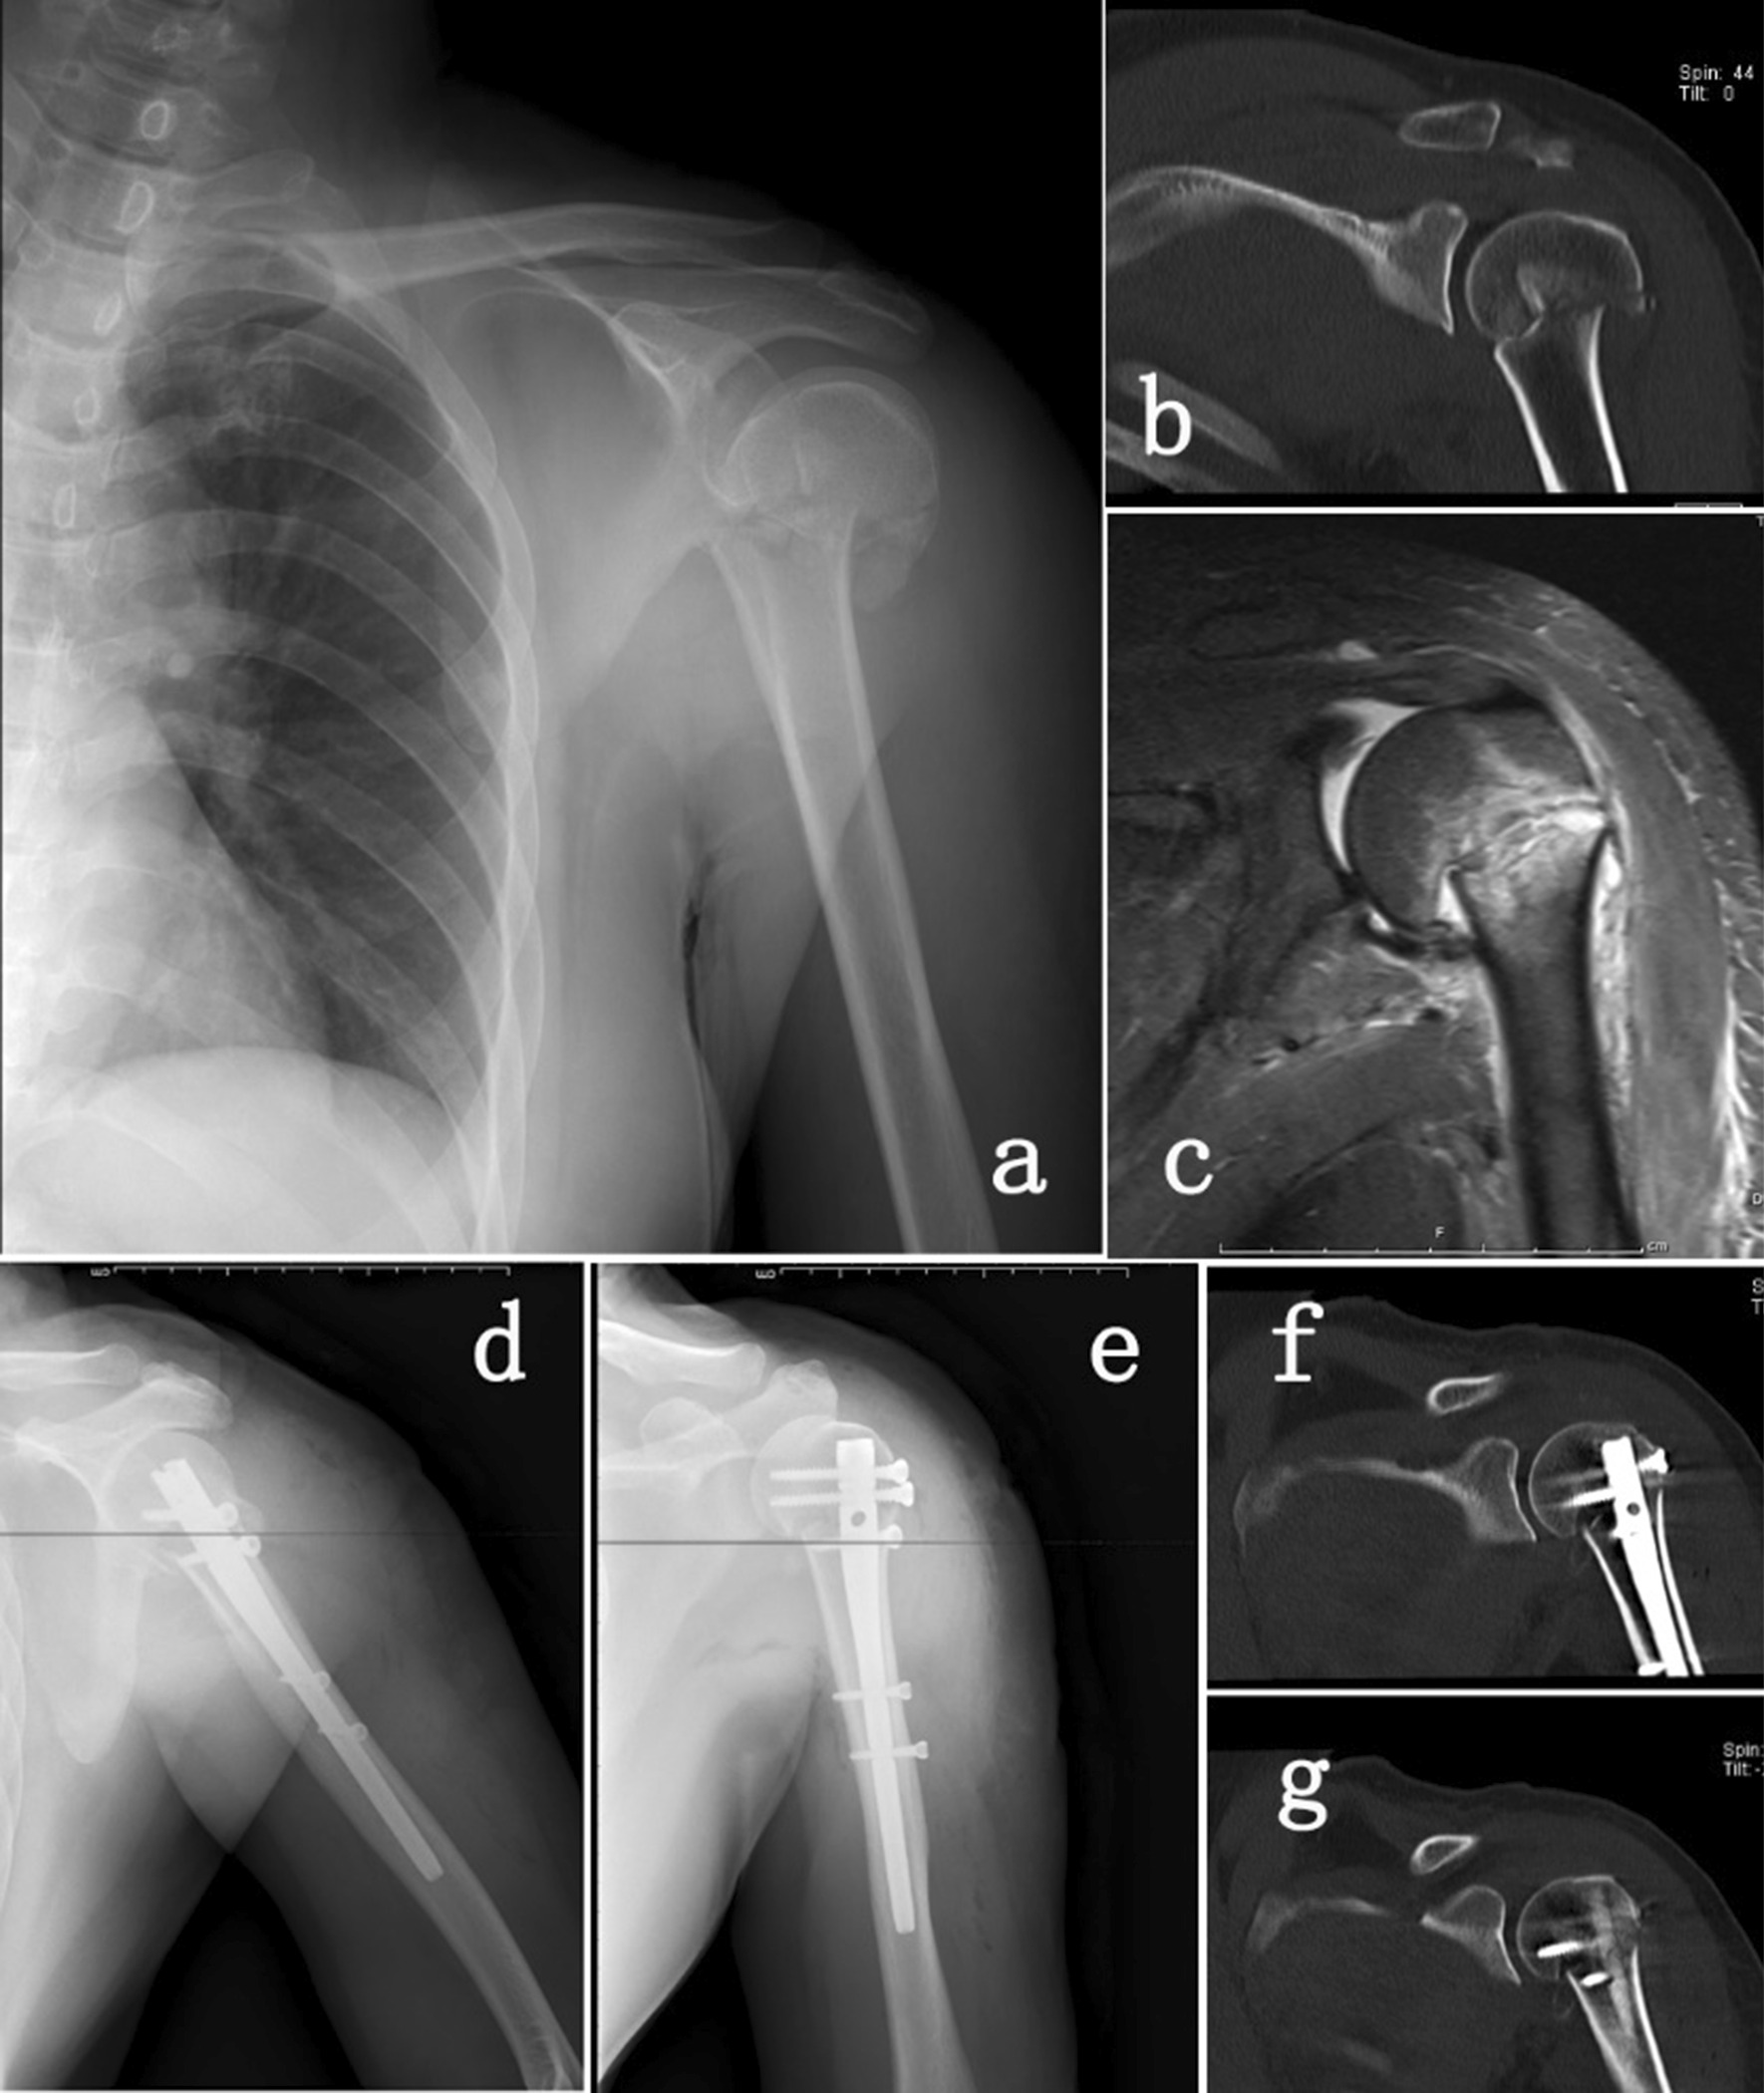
Patients with PHFs were treated with the interlocking intramedullary nail approach. a Preoperative X-ray of the shoulder joint; b preoperative CT; c Preoperative MRI; d, e Postoperative X-ray; f, g Postoperative CT scan of the shoulder joint
